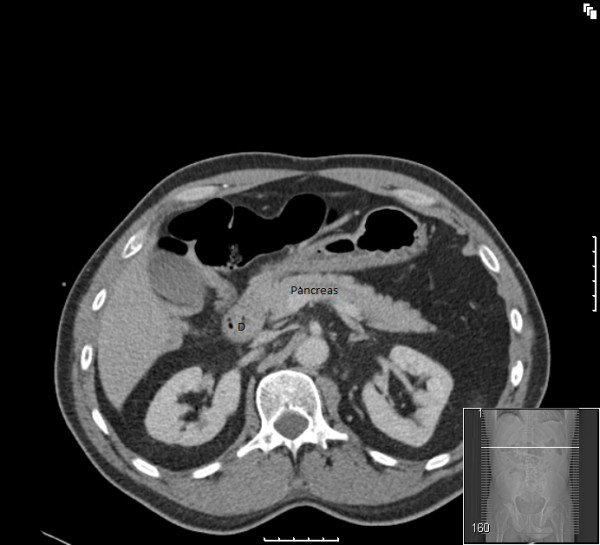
CT scan showing lack of progression of the duodenum across the aorta and the spines (D-duodenum).
